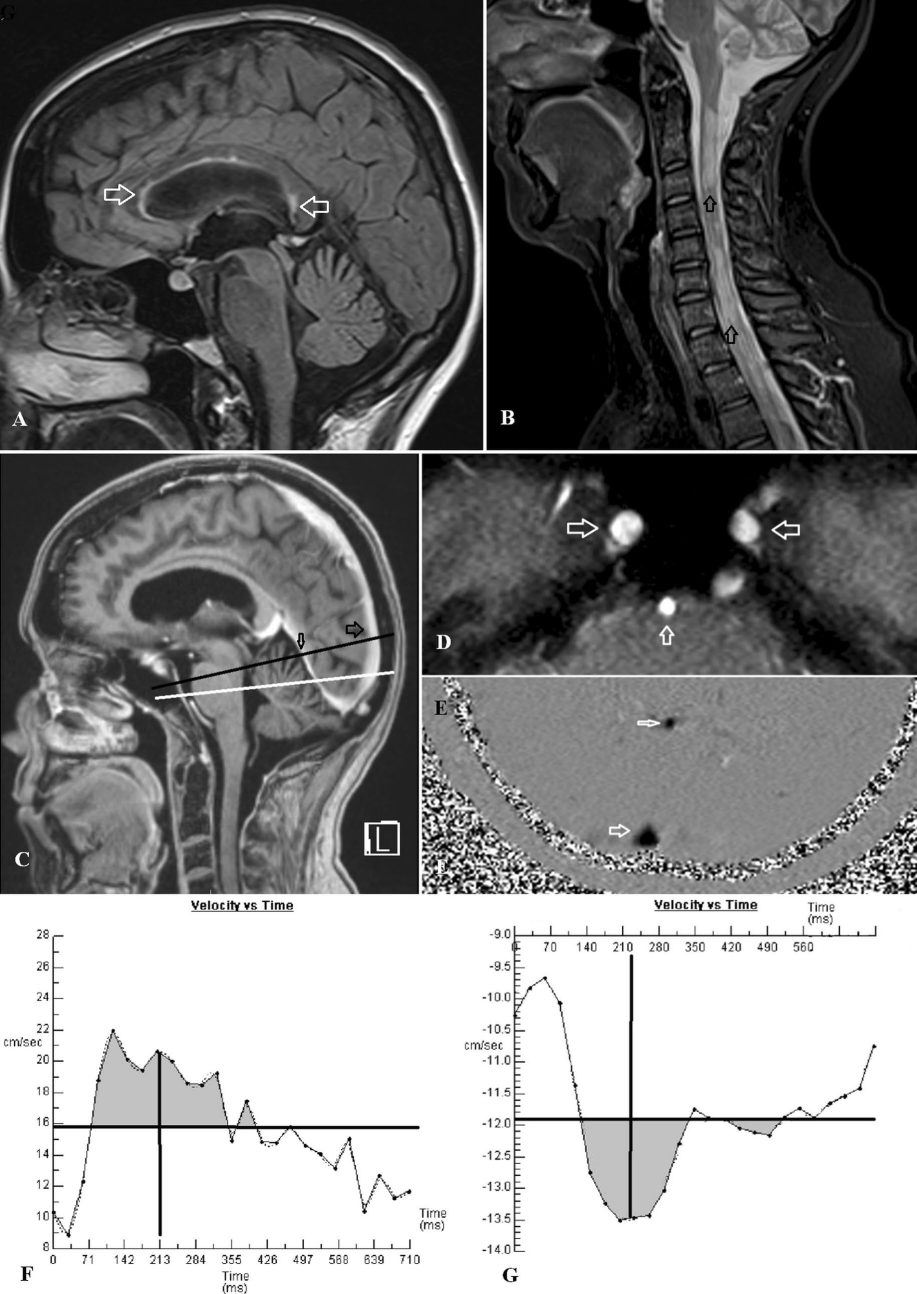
A A FLAIR sagittal image of a 48 year old female MS patient with *arrows* showing some plaques within the corpus callosum but no significant cerebral volume loss. B A T2 sagittal image of the cervical spine in the same patient with arrows showing extensive MS plaques. C A sagittal post contrast T1 image with the *black line* showing the position of the venous acquisition passing through the sagittal sinus (*large arrow*) and straight sinus (*small arrow*). The *white line* is the arterial acquisition passing through the skull base. D The localizer image from the arterial acquisition showing the carotid arteries just above foramen lacerum (*horizontal arrows*) and the mid basilar artery (*vertical arrow*). E The phase image from the venous acquisition showing the sagittal sinus (*large arrow*) and straight sinus (*small arrow*). F The arterial flow graph from the same patient. The *horizontal line* represents the mean flow velocity. Where the mean velocity transects the flow graph is the start and end of systole. The *grey area* above the *line* represents the arterial stroke volume which for the three arteries combined was 1184 µL. The *vertical line* represents the midpoint of the two transection points and is the midpoint of the primary arterial wave i.e. 213 mS. G The sagittal sinus flow graph from the same patient. The *horizontal line* is the mean flow velocity. The *grey area* is the sinus stroke volume which is 118 µL or 10 % of the arterial stroke volume. The *vertical line* is the midpoint of the two transection points and is the center of the primary pulse wave i.e. 223 mS giving an AVD of 10 mS in this patient which is less than 10 % of normal indicating very low compliance
